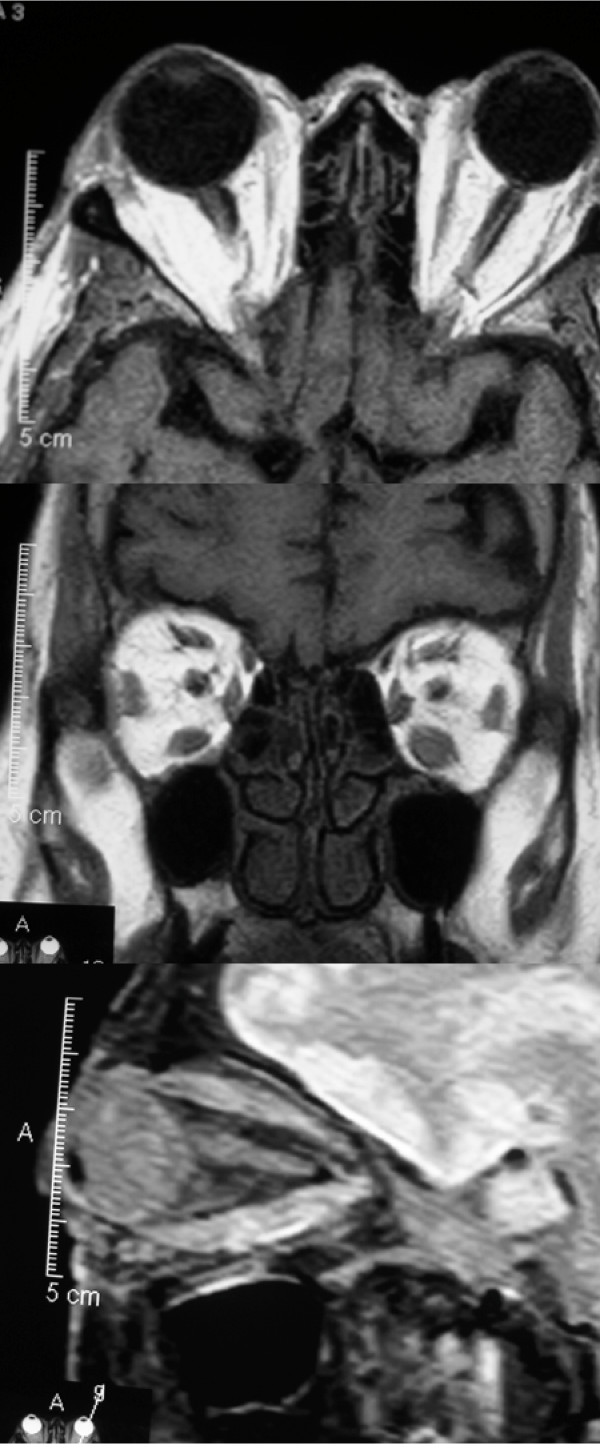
Magnetic resonance imaging scans of patient demonstrating expansion of the orbital fat compartment and enlargement of the left inferior rectus muscle.
